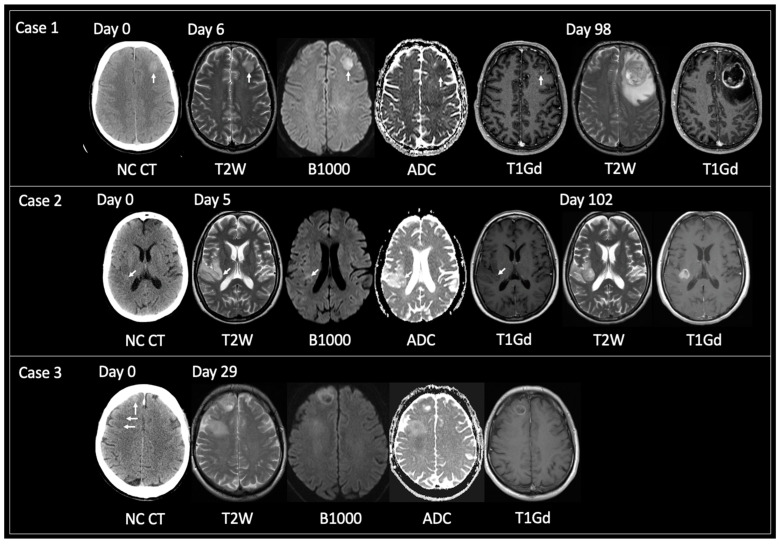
Examples of solitary lesions with TRA GBM at tissue diagnosis that had preceding imaging. Case 1: 56-year-old male presented with seizures. Unenhanced CT on day 0 showed a hyperdense lesion in the left frontal lobe. This had corresponding T2 iso signal with reduced diffusivity and central nodular enhancement on MRI day 6. TRA GBM followed on MRI day 98. Case 2*:* 53-year-old female presented with several days of intermittent left-sided anesthesia. Initial unenhanced CT day 0 showed a focus of hyperdensity within the posterior aspect of the right insula (arrow) with adjacent cortical low density. MRI day 5 revealed an infiltrative glioma within the right inferior parietal lobule and right insular with the focus of prior CT hyperdensity corresponding to T2 iso signal, reduced diffusivity and containing a tiny dot of enhancement (arrows). MRI day 102 showed TRA GBM at the site of the prior CT hyperdense focus but relative stability of the rest of the non-enhancing tumor. Case 3: 63-year-old male presented with seizures. Unenhanced CT day 0 revealed two abnormal areas: a hyperdense focus anteriorly in the right superior frontal gyrus (vertical arrow) and subcortical hypodensity in the right middle frontal gyrus (horizontal arrows). These foci were linked by hypodensity (not shown) correlating with one diffuse tumor. MRI day 29 demonstrated interval growth of the previously hyperdense lesion, showing TRA GBM with enhancing periphery and central necrosis. Note how the peripheral tumoral tissue shows T2 iso solid signal with reduced diffusivity and enhancement. MRI day 29 also showed progression of the previously low-density lesion but not to TRA GBM. This lesion was shown to infiltrate into the deep white matter of the right centrum semiovale, contain areas of reduced diffusivity but no enhancement. TRA GBM—typical radiological appearance of glioblastoma, NC CT—non-contrast CT, T2W—T2-weighted, B1000—DWI, ADC—apparent diffusion coefficient map of DWI, T1Gd—T1-weighted post-gadolinium.
